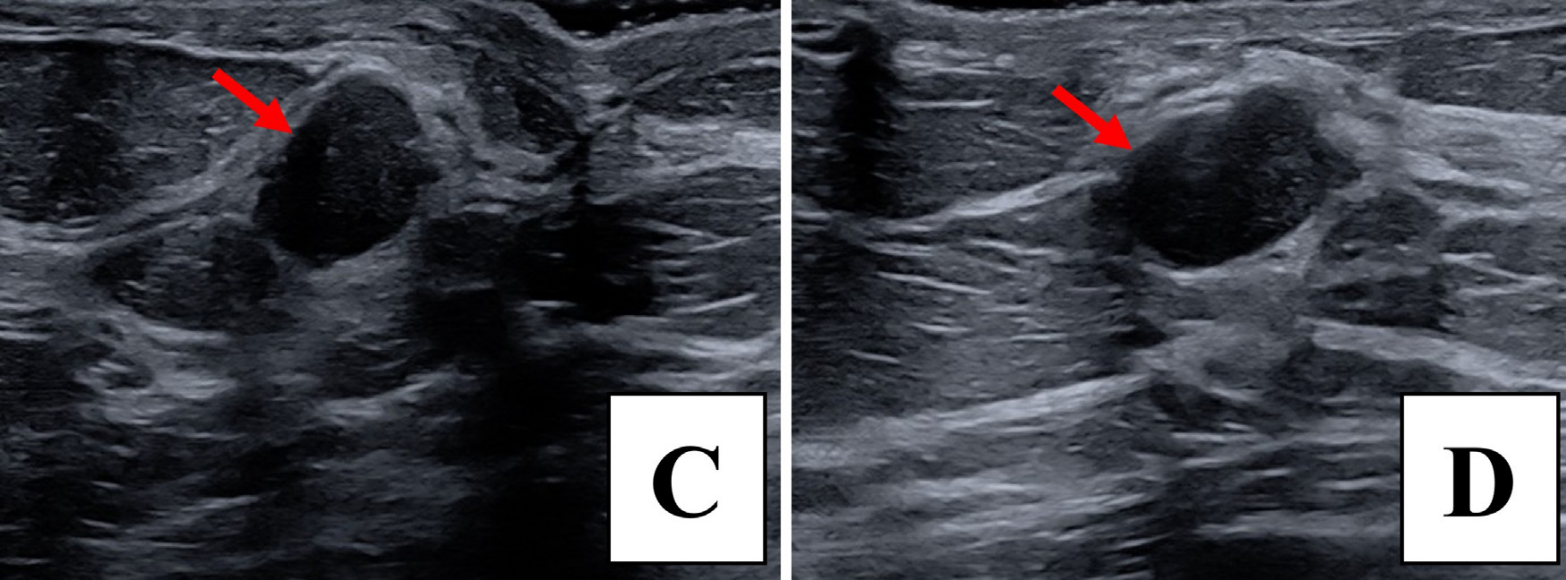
In the left retro-areolar region, ultrasound reveals an oval, parallel, hypoechoic 1.6 × 1.2 × 1.0 cm mass with angular margins in sagittal (C) and transverse (D) views, with red arrows highlighting the lesion.
